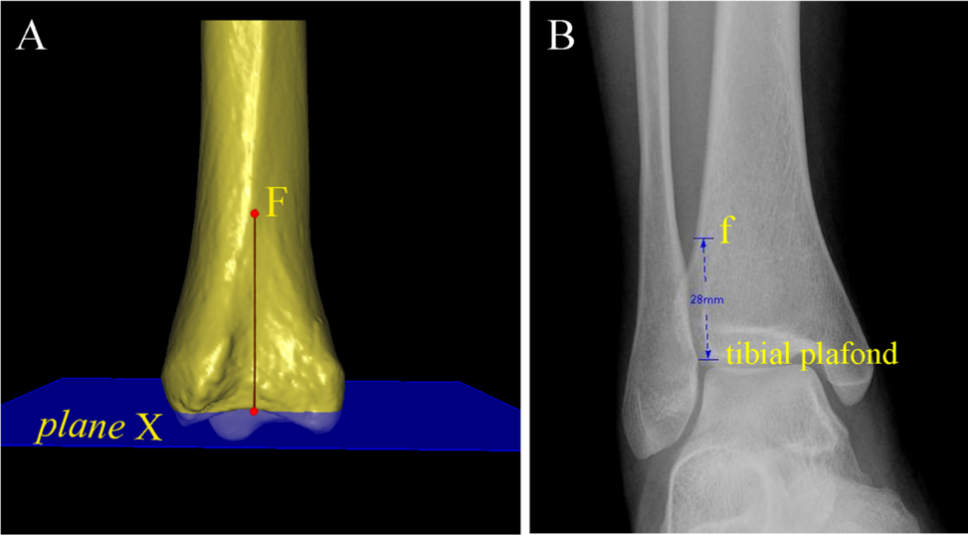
The measurement of the height of incisura fibularis was carried out. a The proximal tip of incisura fibularis was selected as “point F”, and the vertical distance from the point F to plane X in the 3-D image was measured. b The height of incisura fibularis in the AP view radiograph was described as the perpendicular distance from the proximal tip of incisura fibularis to the tibial plafond
